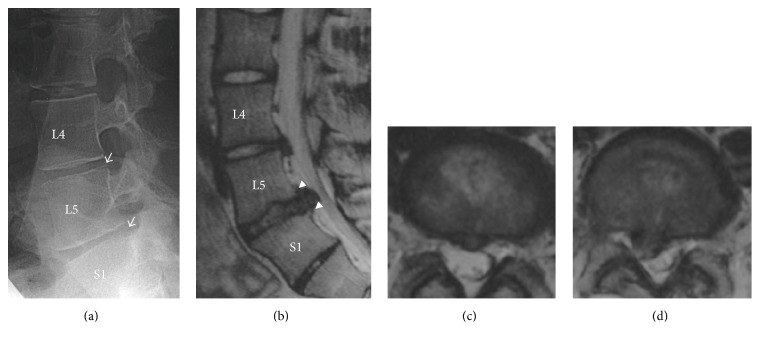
Radiographs showed lumbar posterior endplate lesions at L4/5 and L5/S1 at 14 years of age ((a), white arrows). Sagittal (b) and axial ((c) and (d)) T2-weighted MR images revealed L4/5 and L5/S1 lumbar disc herniation with endplate lesion. White arrow head showed irregular shape of the posterior end plate at the corner of S1 and L5 vertebrae.
